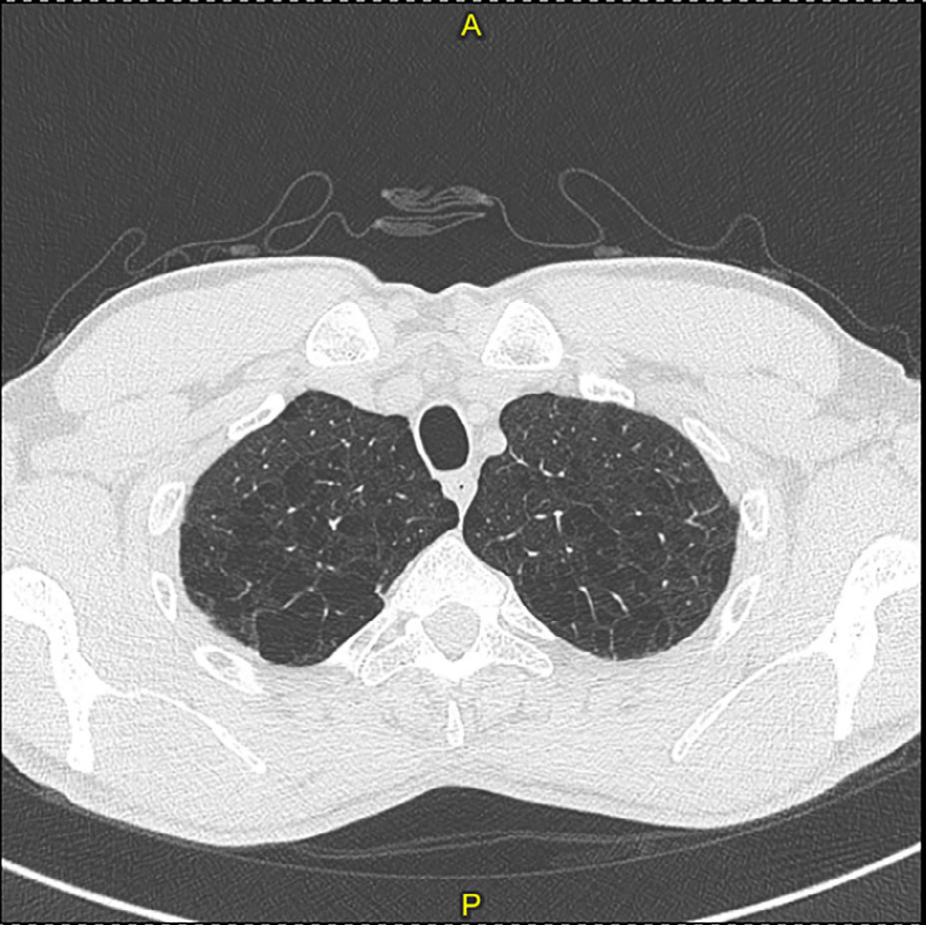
High resolution computed tomography of the Case 3 patient, showing upper lobe panlobular emphysema.
